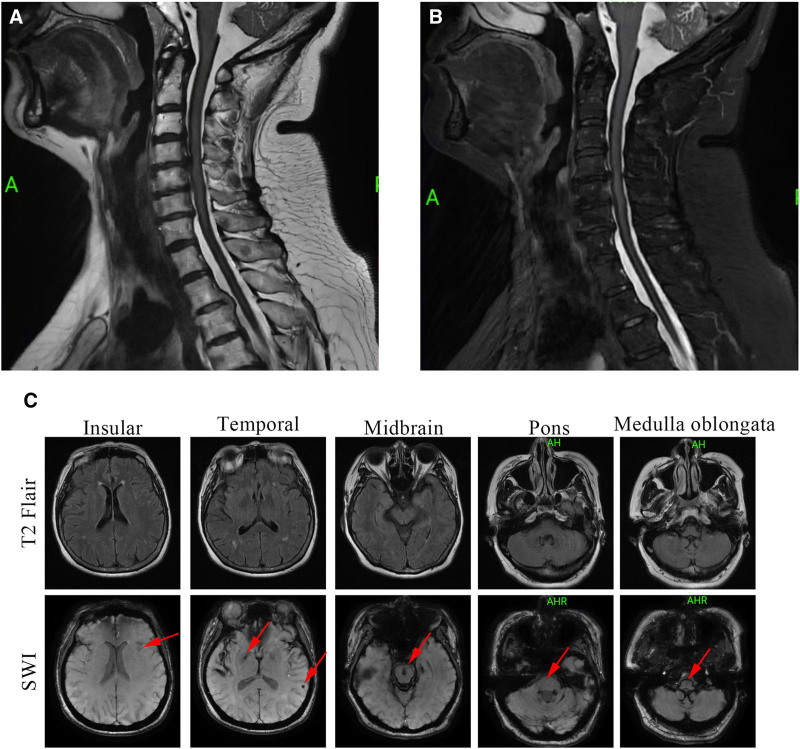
Magnetic resonance imaging shows a toroidal line of low T2 signal on the spinal cord margin on a T2-weighted image, involving the cervical spinal cord (A, B). Brain T2-weighted MRI does not clearly show hemosiderin deposition. Brain MRI using sensitivity weighted imaging (SWI) shows hemosiderin deposits in the bilateral insula, temporal cortex sulci walking area, and around the brain stem (arrows) (C).
